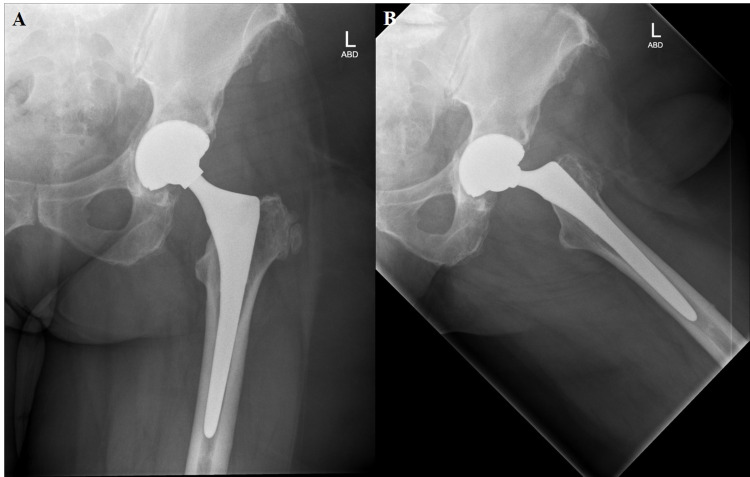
Nine-month postoperative anterior-posterior (A) and lateral (B) radiographs with signs of cup loosening. There is medial wall fragmentation and cystic change around the greater trochanter.
